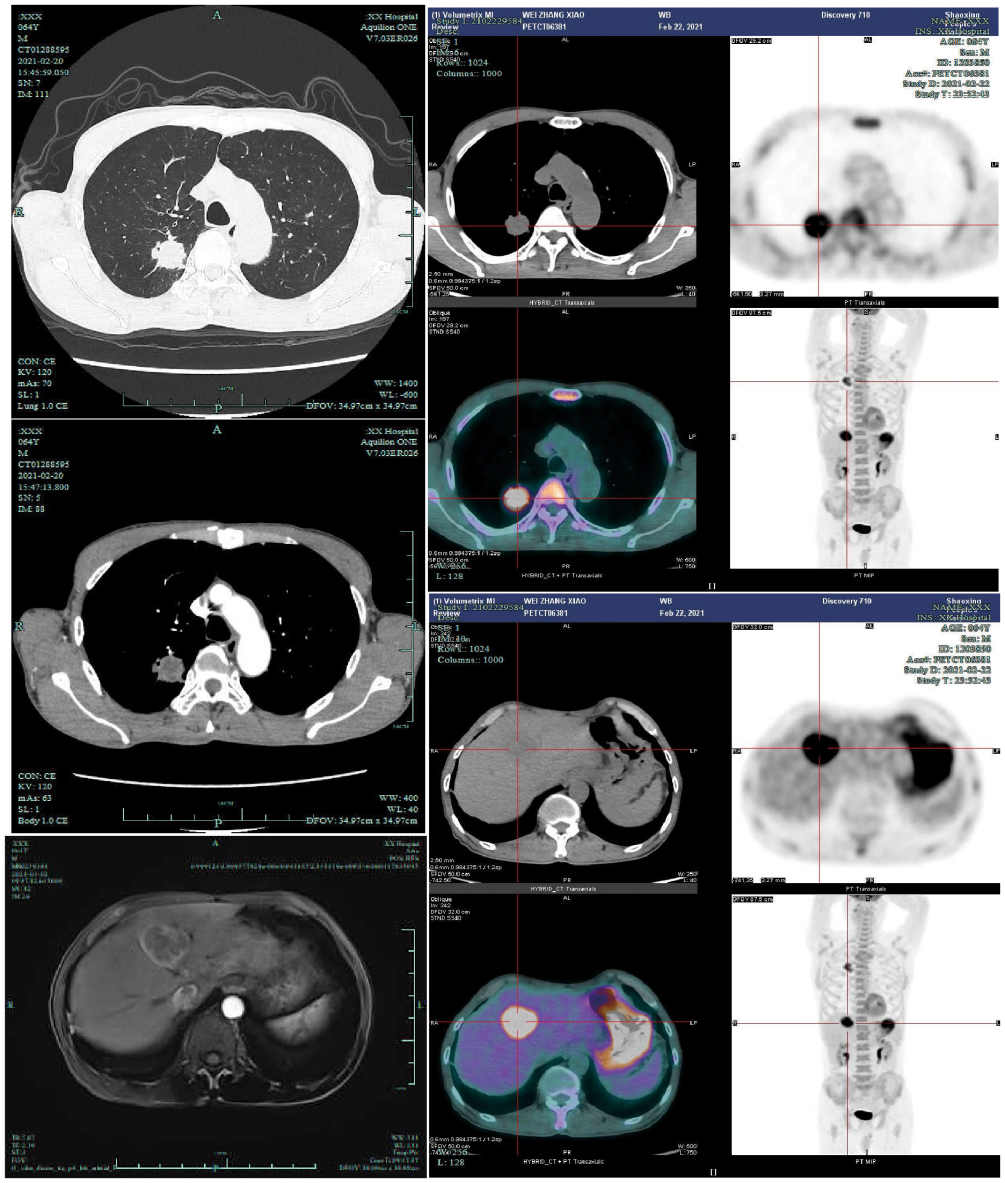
Upon admission, the patient underwent contrast-enhanced chest CT(2021-2-20), contrast-enhanced liver MRI(2021-3-2), and PET-CT examinations(2021-2-23). PET-CT demonstrated a right upper lung nodule with a maximum standardized uptake value (SUVmax) of 8.30, and a liver mass with SUVmax of 11.89 on conventional imaging and 13.00 on delayed-phase imaging.
